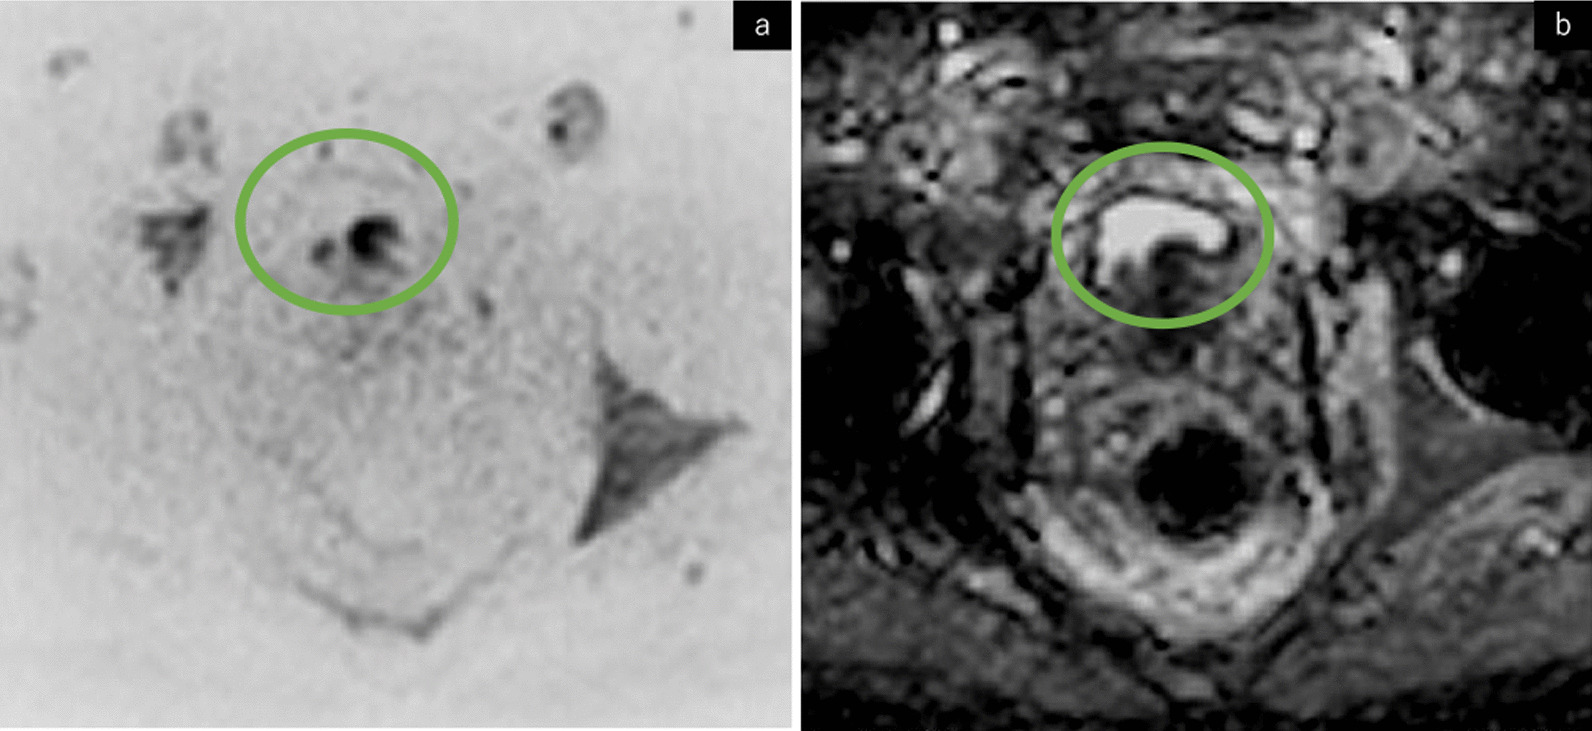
Magnetic resonance imaging showing the central to transitional area of the prostate protruding toward the bladder, and diffusion-weighted images (a) and apparent diffusion coefficient (b) mapping showing diffusion disorders (green circle)
